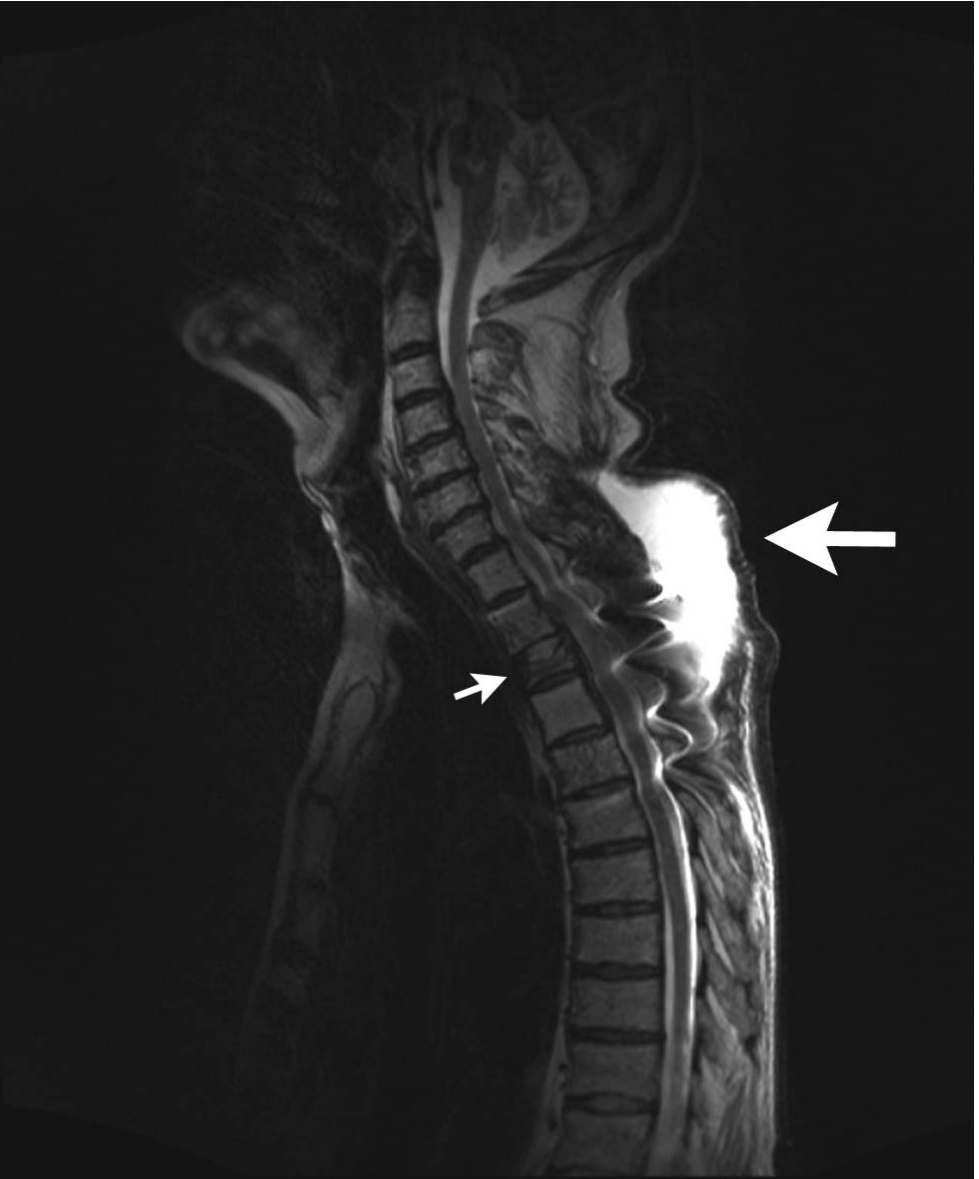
MRI of the thoracic spine (T2-weighted, sagittal reconstruction). The dorsal fluid collection suggests a paravertebral abscess (large arrow) just above the fractured and operated third thoracic vertebra (small arrow).
